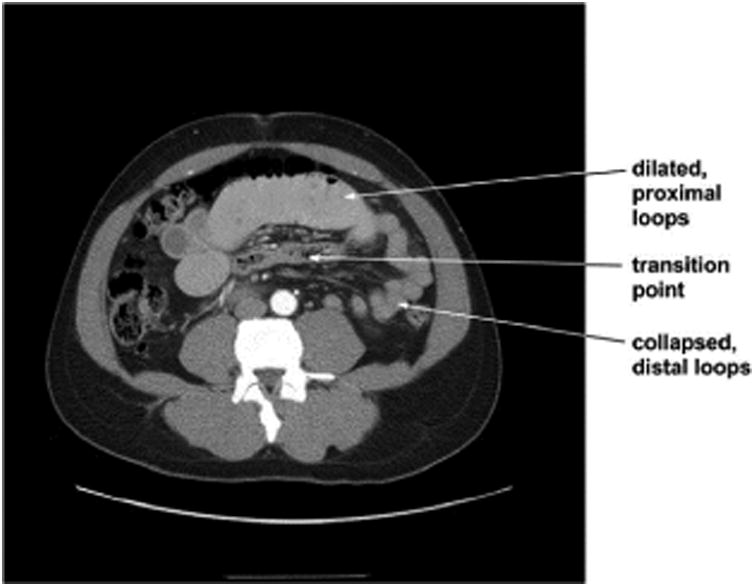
CT of patient revealing dilated loops of small bowel (proximal) and collapsed loops of small bowel (distal).
